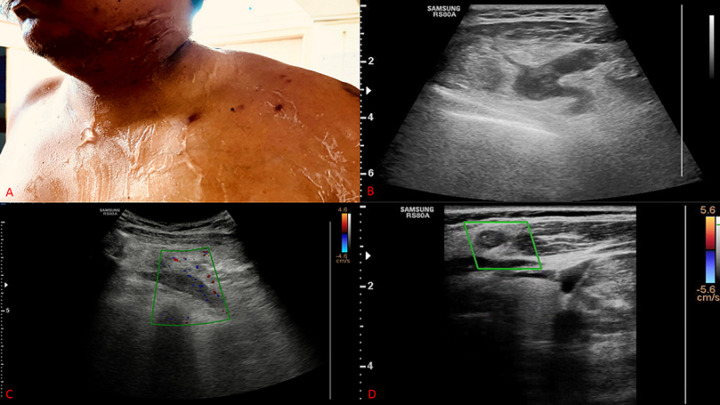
A) swelling and redness in neck region; B) B-mode ultrasonography image in longitudinal section showing complete thrombosis of external jugular vein; C) color Doppler image showing no flow in external jugular vein in longitudinal section; D) color Doppler image showing no flow in external jugular vein in transverse section
